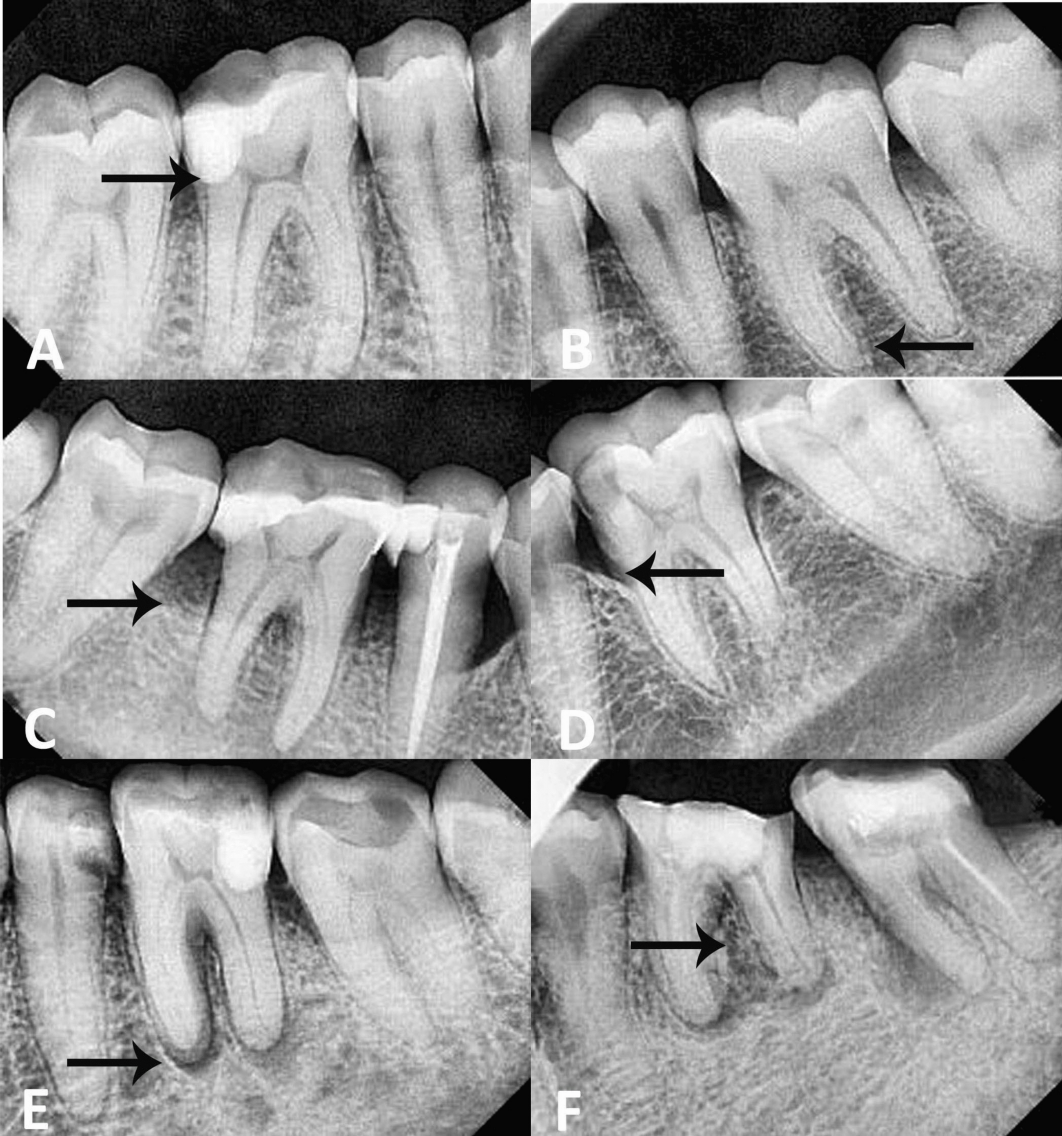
Types of endo-perio lesions (A) type 1 endo lesion, (B) type 3 perio lesion, (C) type 2 (primary endo and secondary perio lesion) with horizontal bone loss, (D) type 4 (primary perio and secondary endo lesion) with vertical bone loss, (E) type 2 lesion with periapical pathology, and (F) type 4 lesion with periodontal abscess.
